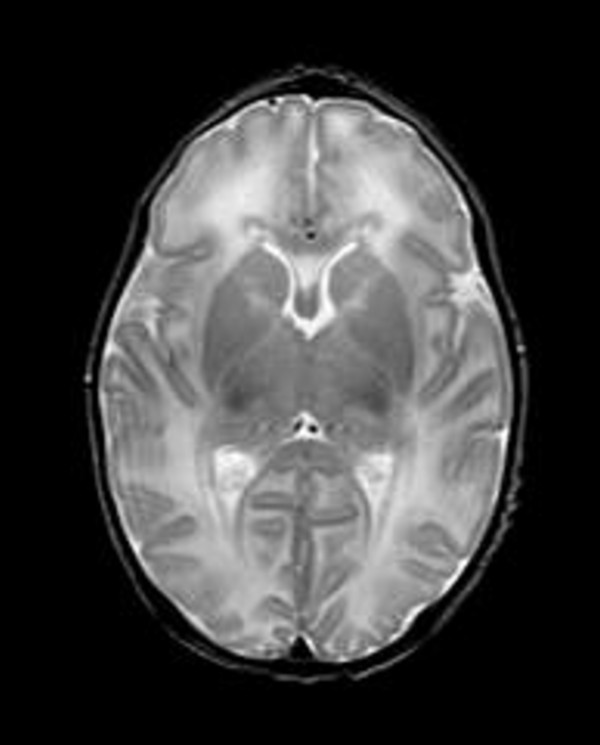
Cerebral MRI (whitened T2 sequences), showing diffuse white matter periventricular hyperintensity (hypointensity in T1 sequences).
